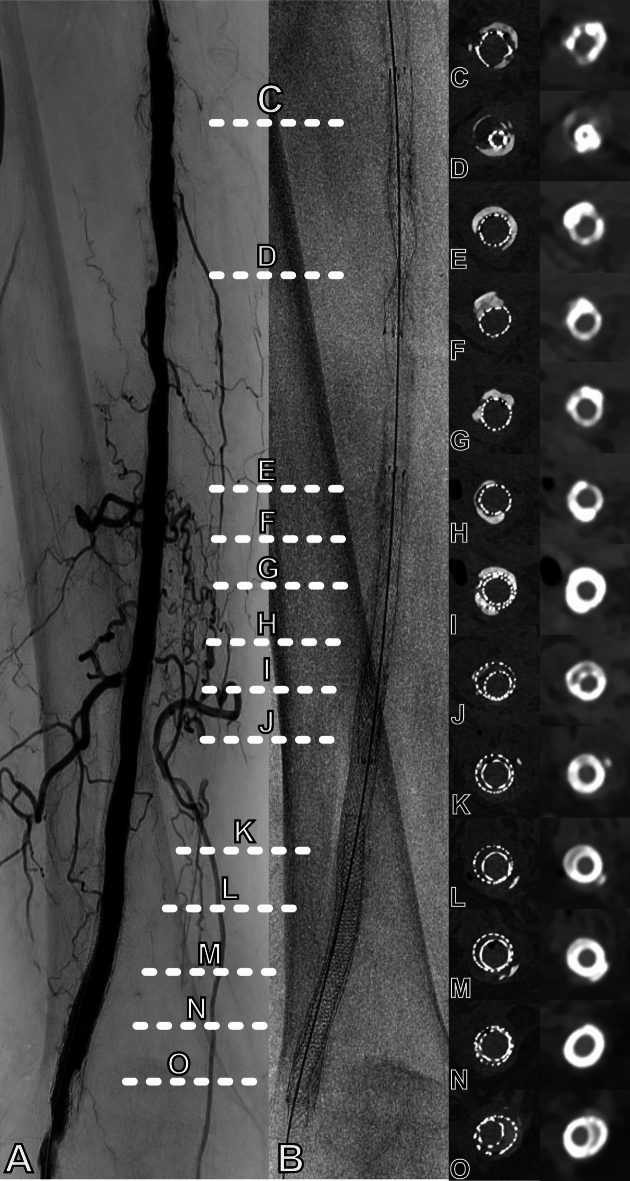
Different advanced stage peripheral arterial disease scenarios. (A) Digital subtraction angiography of the right upper leg after stent deployment with several stenoses; (B) corresponding fluoroscopy with depiction of stents and heavy calcifications; C–O: Axial slices of the evaluated scenarios with stent underexpansion (C), severe stent underexpansion (D), stent true-to-size with concurrent severe calcification (E, G, H), stent true-to-size with eccentric compression by severe calcification (F), stent-in-stent with inner stent true-to-size and severe calcification (I), stent-in-stent with underexpansion of the inner stent (J–L, O), and stent-in-stent with the inner stent true-to-size without relevant calcifications (M, N). Left column depicts the maximum-dose reference scan, while right column depicts images from standard-resolution PCD-CT (BV40) at 3 mGy. White dotted lines indicate the levels of measurements.
